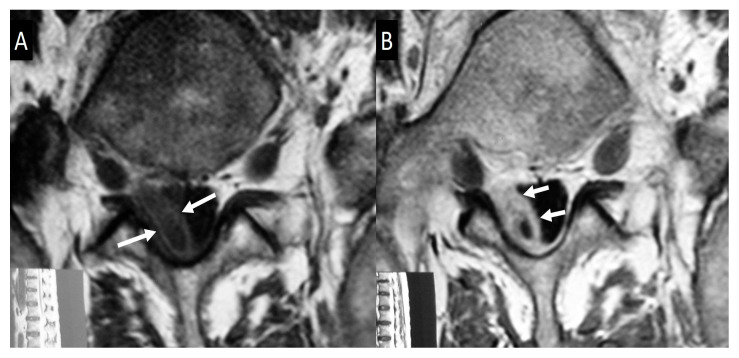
Axial plain (A) and contrast-enhanced (B) T1-w MR images, showing the epidural abscess formation on the right side (arrows in A), with wall enhancement (arrows in B), and displacement of the dural sac to the left.
